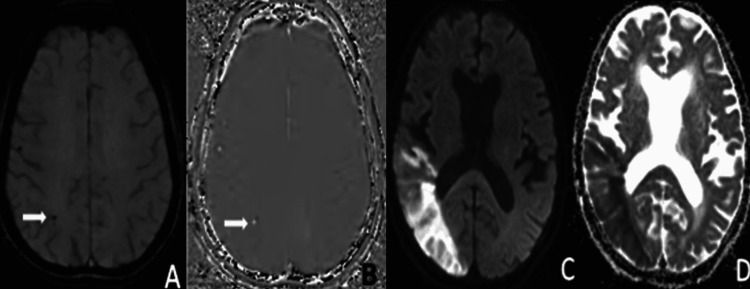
A well-defined rounded hypointense area on magnitude image (A) appearing hyperintense on phase (B) in seen in the right occipital region (white arrow) suggestive of microhaemorrhage. A large area of diffusion restriction on DWI (C) and corresponding low ADC (D) in seen in the right parieto-occipital region, suggestive of right posterior MCA territory acute infarct with microhaemorrhage within infarcted area.
